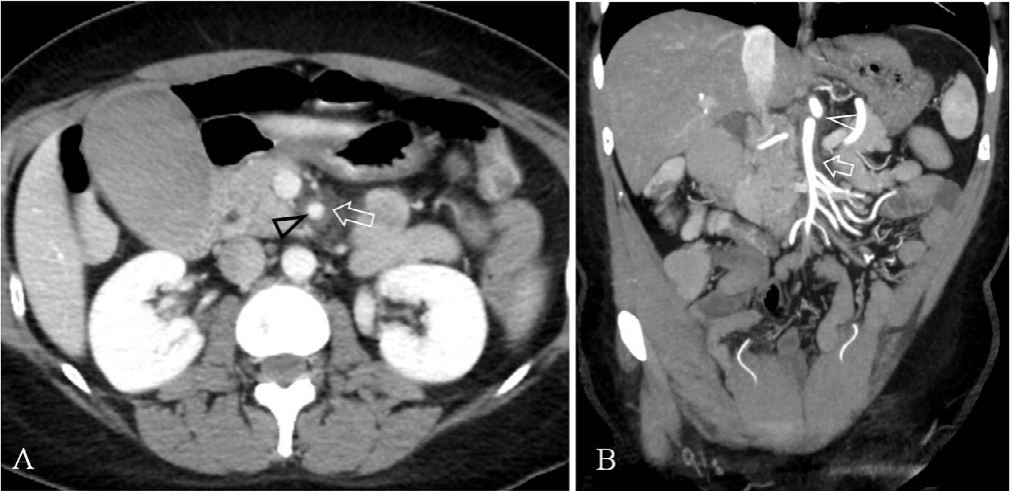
CT scan following intravenous contrast administration, portal phase. (A) Axial slice shows the enhancing superior mesenteric artery (arrowhead) with an intact smooth lumen, without evidence of dissection. Note perivascular fat stranding as circumferential increased density of adjacent fat (arrow). (B) Maximum intensity projection reconstruction in the coronal plane shows the extent of perivascular fat stranding (arrow) which was appreciated as progressive compared to the initial scan and situated around the normal lumen of superior mesenteric artery and adjacent celiac artery (arrowhead).
